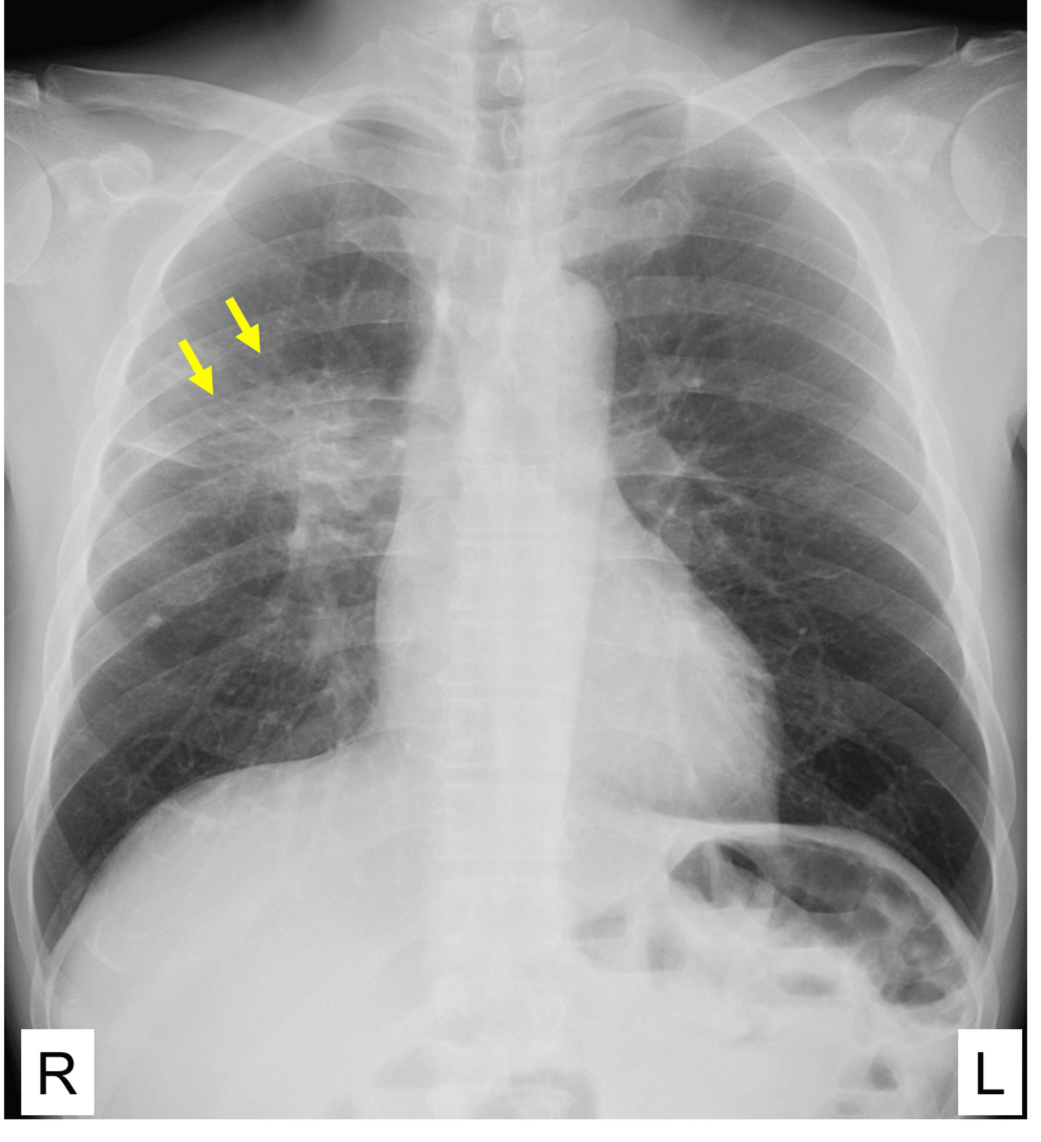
Chest X-ray of the chest at the first visit An abnormal nodular shadow was observed in the right middle lung field, along with prominence of the right hilar region, and lung consolidation in the lower part of the right hilus. R: right L: left.
